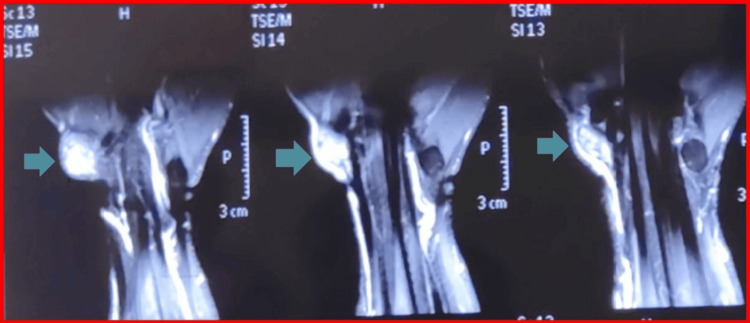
T2-weighted serial coronal MRI sections of the wrist These coronal MRI sections show the tumor displacing the radial artery laterally, both appearing as T2 hyperintense, with hypointense areas suggestive of hemosiderin deposits and blooming artifact on the MRI sections, and the FCR tendon appearing isointense. FCR: flexor carpi radialis; MRI: magnetic resonance imaging
